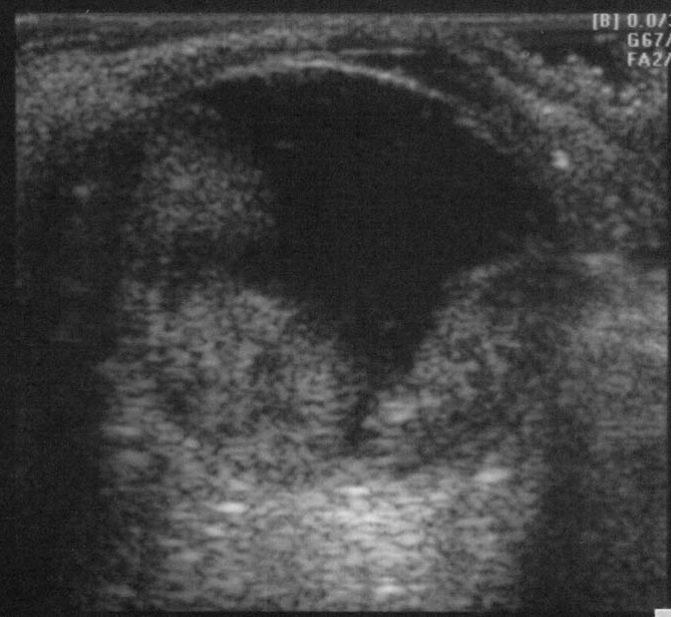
B-scan ultrasonography of the left eye at presentation.
